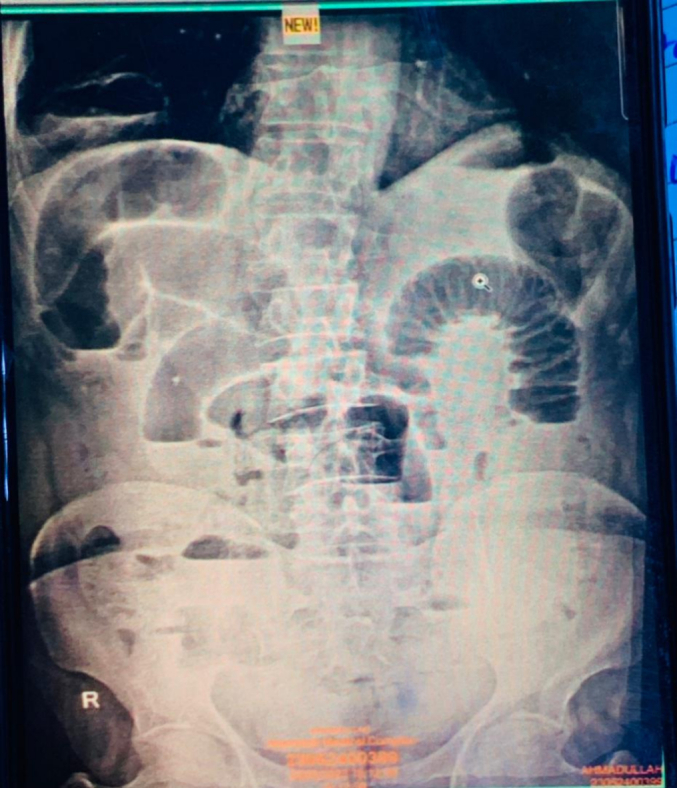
Radiograph of the abdomen and lower part of the chest in a standing position showing dilated bowel loops, and multiple air-fluid levels suggestive of small bowel obstruction. A loop of small bowel is also seen inside the chest above the right hemi-diaphragm.
